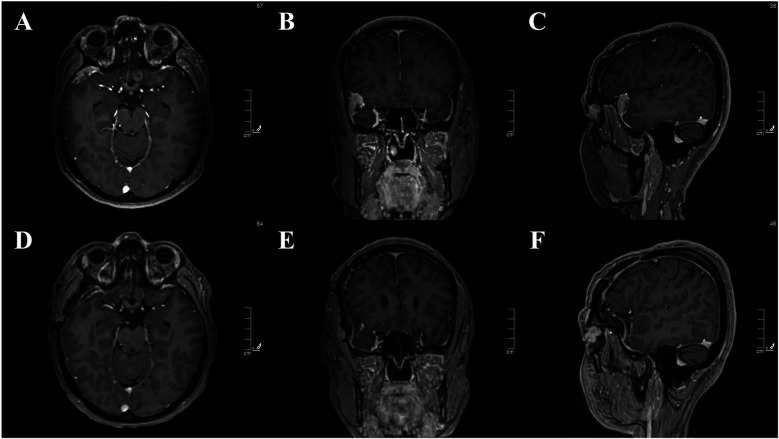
(A–C) Pre-operative contrast-enhanced MRI images of a solitary leptomeningeal dissemination of an previously gross total resected and irradiated aCN in the right sylvian fissure, atteched to the lateral sphenoid wing. (D–F) Post-operative contrast-enhanced MRI images of gross total resection.
